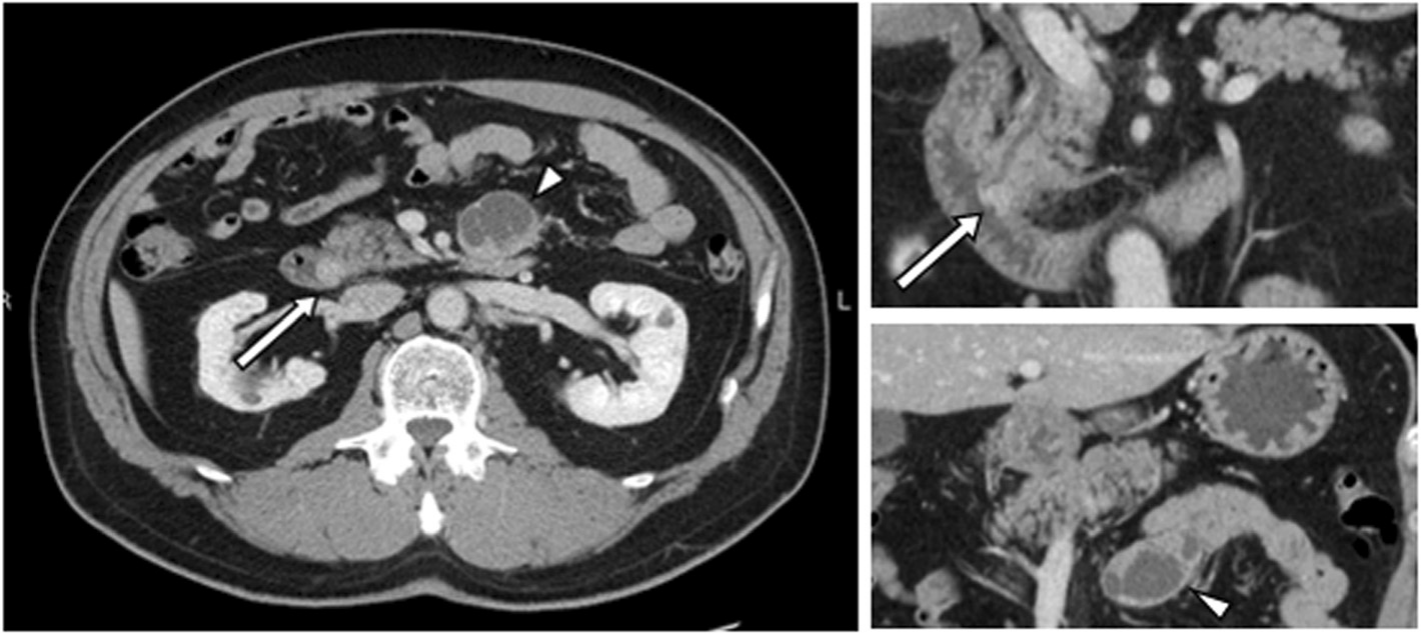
Enhanced abdominal computed tomography scan shows a 10-mm hypervascular tumor at the ampulla of Vater (*arrow*) and a 41-mm multilocular cyst adjacent to the wall of the jejunum near the ligament of Treitz (*arrowhead*)
